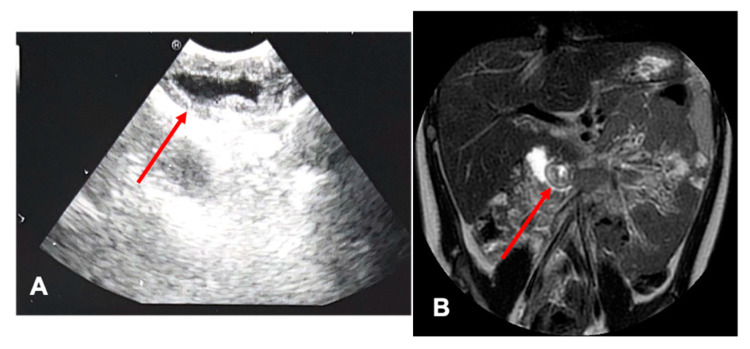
(A,B) Endoscopic US (A) and MRI (B) evaluation. Duodenal duplication in patient n. 7 (red arrows) with a cystic lumen surrounded by multiple layers, well evident on endoscopic US scan.
